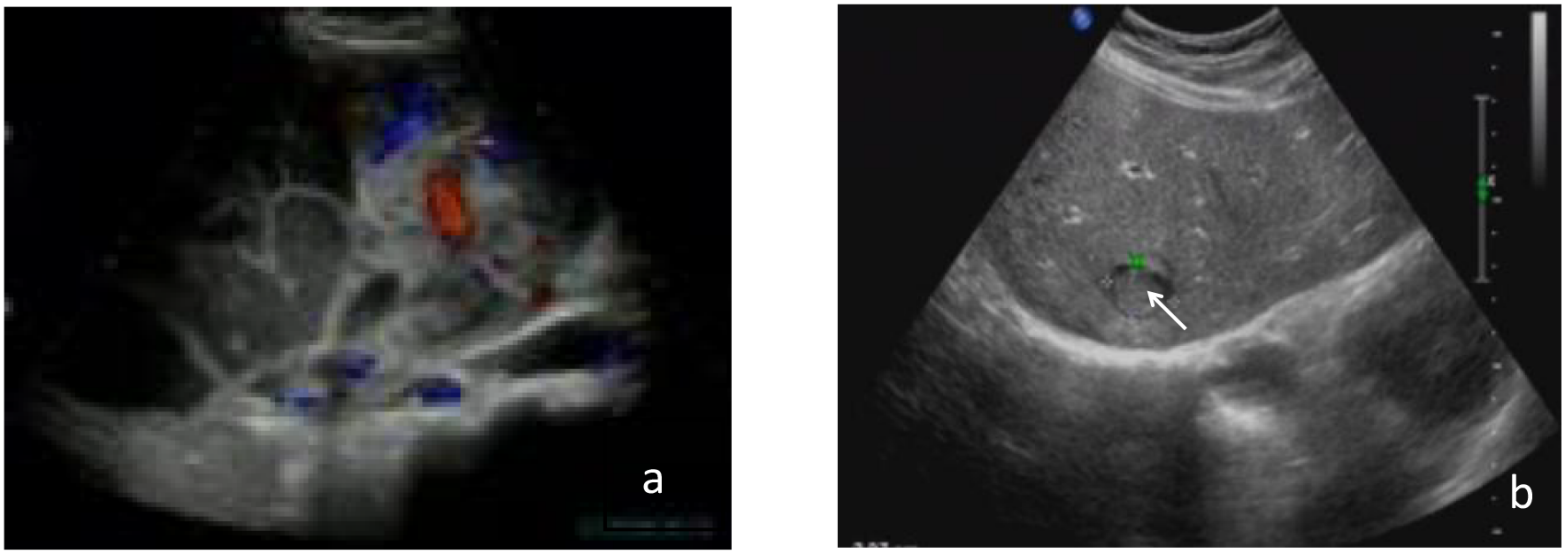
Ultrasonography of PHNEN. (a) Cystic-solid mass with internal septations, poor sound transmission, dense punctate echoes, and blood flow signals in the solid part; (b) Hypoechoic nodule.
